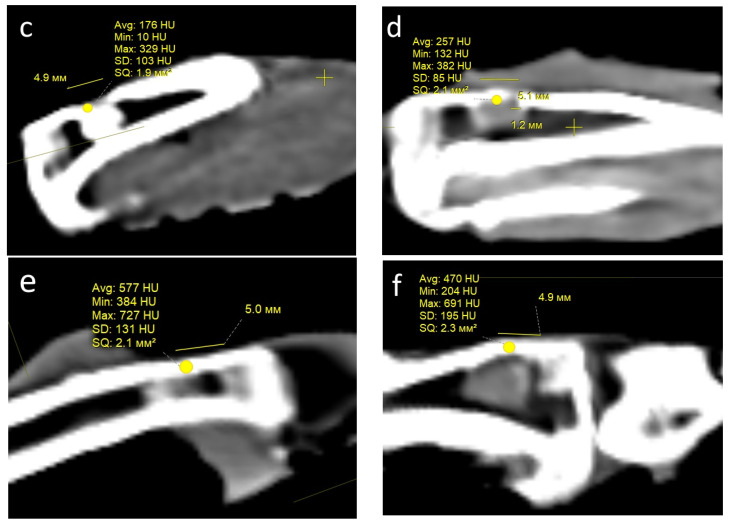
CT scan—section through the center of the implant in the sagittal direction, observed: at 1 month: (a) control, (b) experiment; at 2 months: (c) control, (d) experiment; at 6 months: (e) control; (f) experiment.
